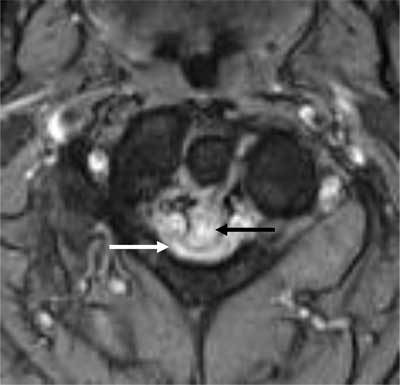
Axial T2-weighted image. The retro-odontoid pseudotumor is seen as a hyperintense mass (black arrow) which extrudes through the transverse ligament and compresses the myelum (white arrow).
